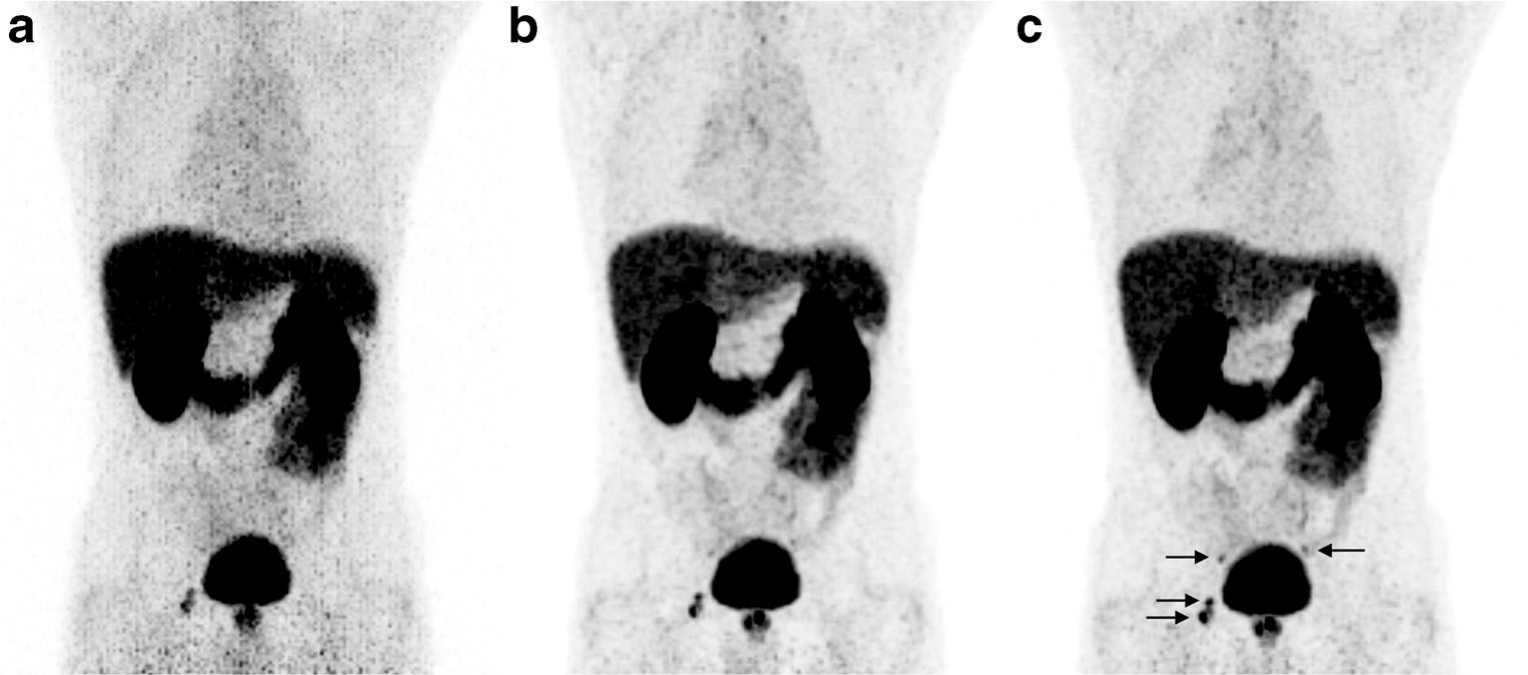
^68^Ga-labeled prostate-specific membrane antigen (PSMA) maximum intensity projection PET images (mCT, Siemens) of a patient with metastasized prostate cancer. PSMA uptake is visible in the prostate and four metastases (two lesions in the acetabulum (*right*), and two para-iliac lymph nodes (*left and right*)). All images were reconstructed with a transaxial matrix size of 256 × 256, pixel size of 3.1 × 3.1 mm^2^. (a) PET reconstruction without PSF modeling and without TOF, (b) PET reconstruction with PSF modeling and without TOF, and (c) a PET reconstruction with both PSF modeling and TOF (data are from Radboudumc, Nijmegen, The Netherlands)
